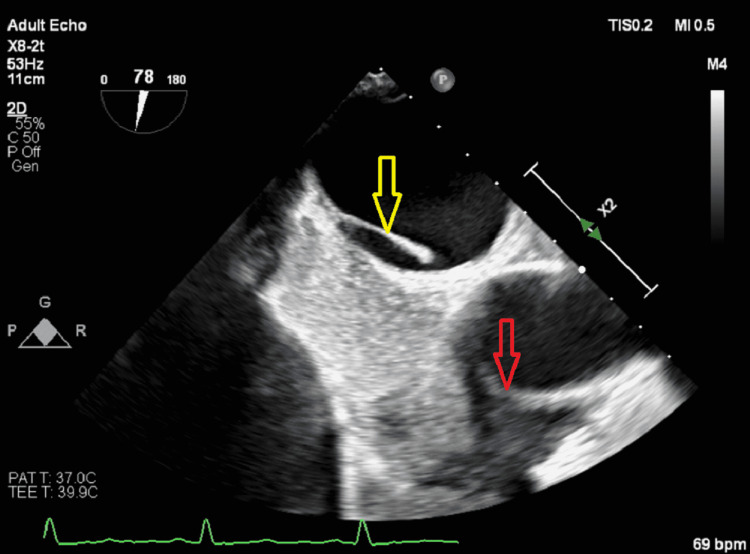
TEE bicaval view with saline agitated study showing a LASP (yellow arrow) and saline bubbles in the right atrium (red arrow) but not crossing into the right atrium TEE: transesophageal echocardiogram; LASP: left atrial septal pouch
